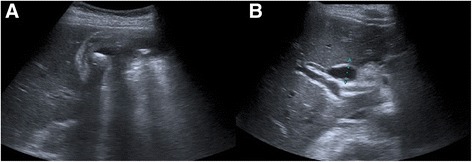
Abdominal ultrasound showing gallbladder wall thickening, pericholecystic fluid and cholelithiasis (a). The common bile duct is dilated (b)
